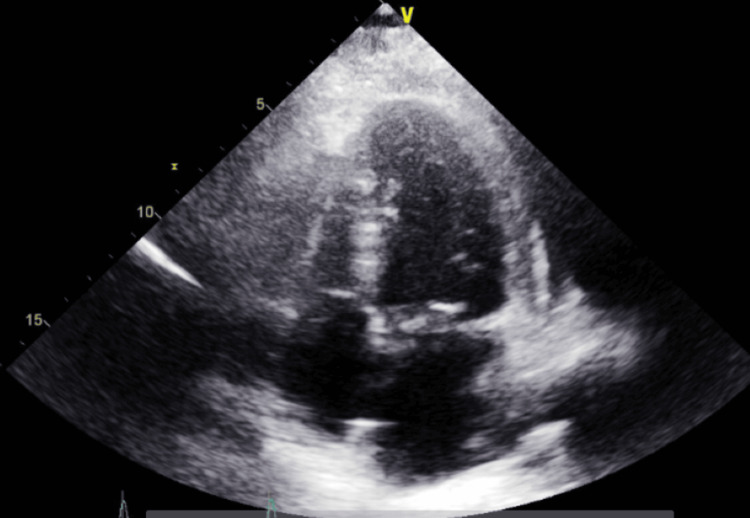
Transthoracic echocardiography - image 3 Transthoracic echocardiography on admission; apical 4-chamber view
